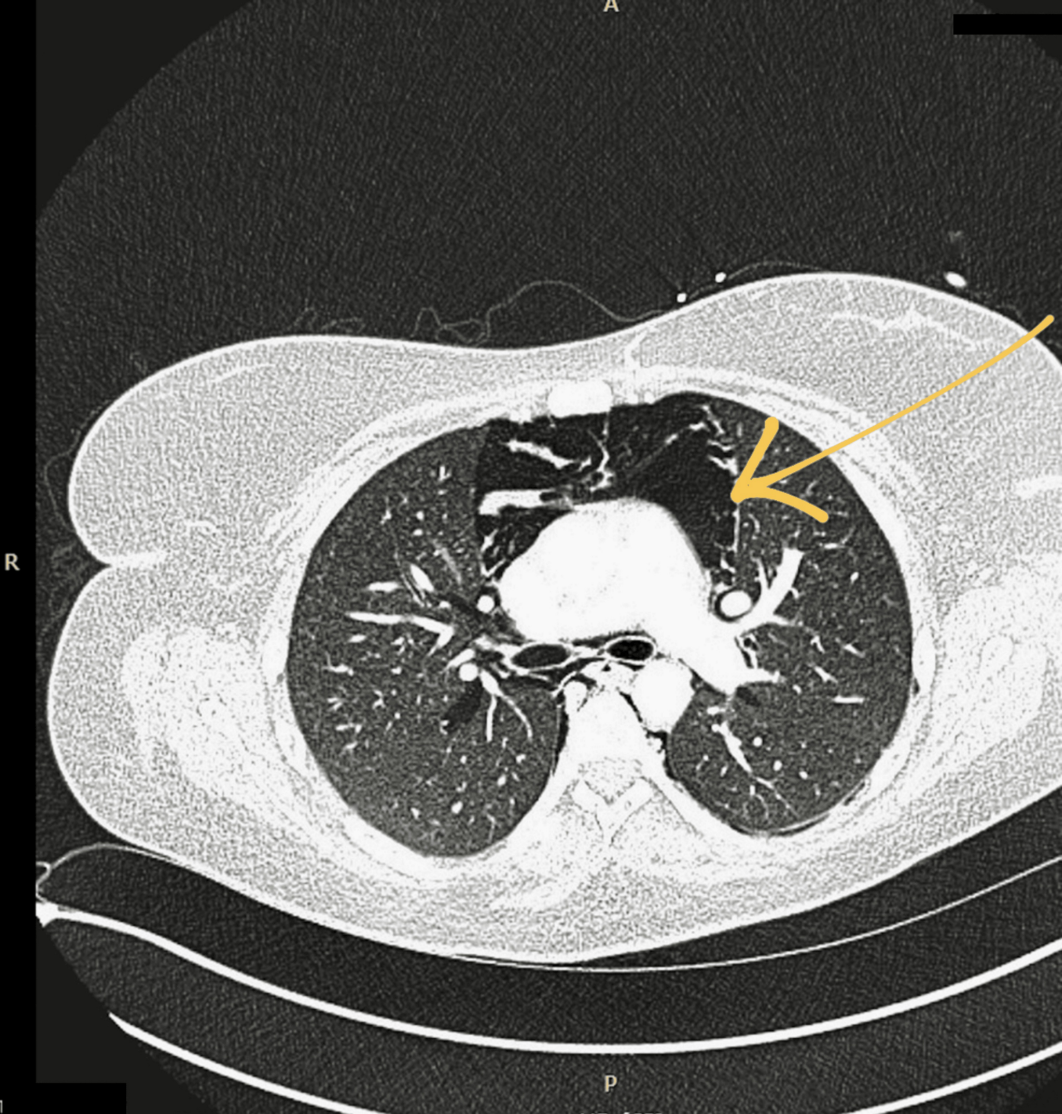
Axial CT image demonstrating pneumomediastinum and air tracking into the neck soft tissues. CT, computed tomography
